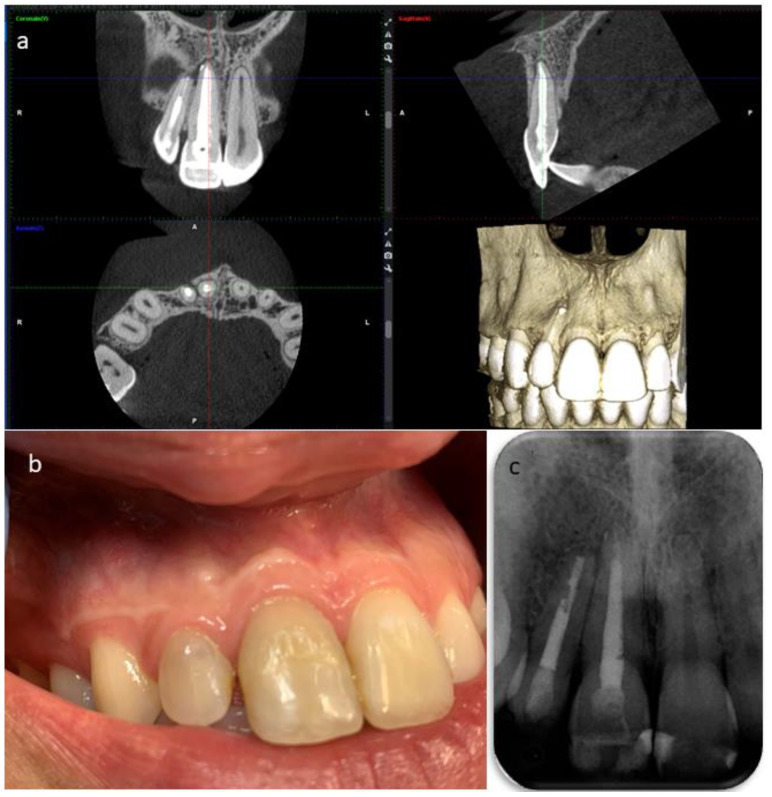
Follow-up at three years. (a) Post-operative CBCT performed at three years. (b) Clinical examination at three years revealing healthy soft tissues at the periapical area. (c) Periapical radiograph taken after three years postoperatively.
